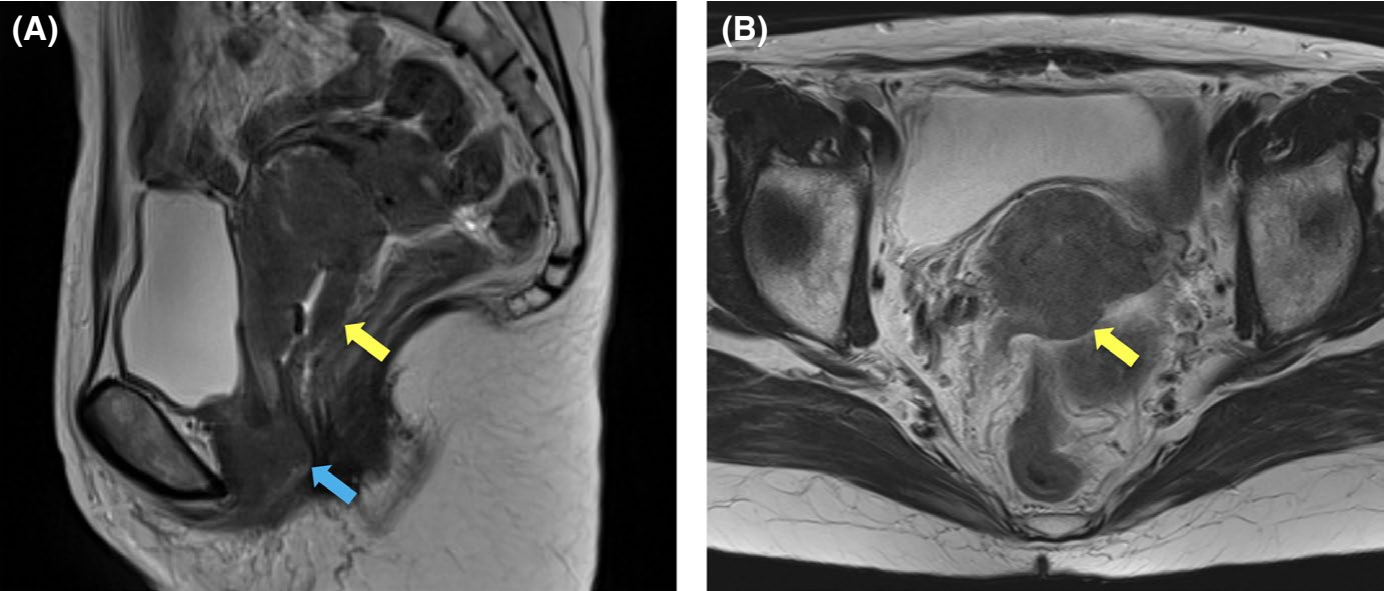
T2‐weighted magnetic resonance images of the primary cervical tumor taken at the time of diagnosis. (A) Sagittal plane. (B) Axial plane. Yellow arrows indicate the primary tumor at the cervix (57 × 48 × 69 mm). A blue arrow indicates the skip lesion in the anterior wall of the lower vagina (39 × 29 × 29 mm)
